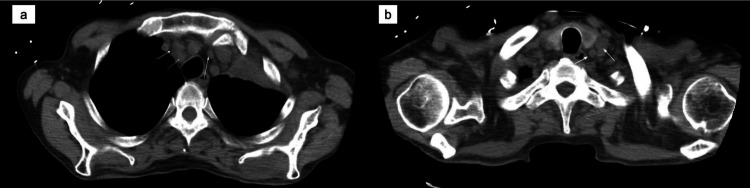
computed tomography CT chest showing mediastinal (a) and left supraclavicular lymphadenopathy(b) which is likely metastatic.
